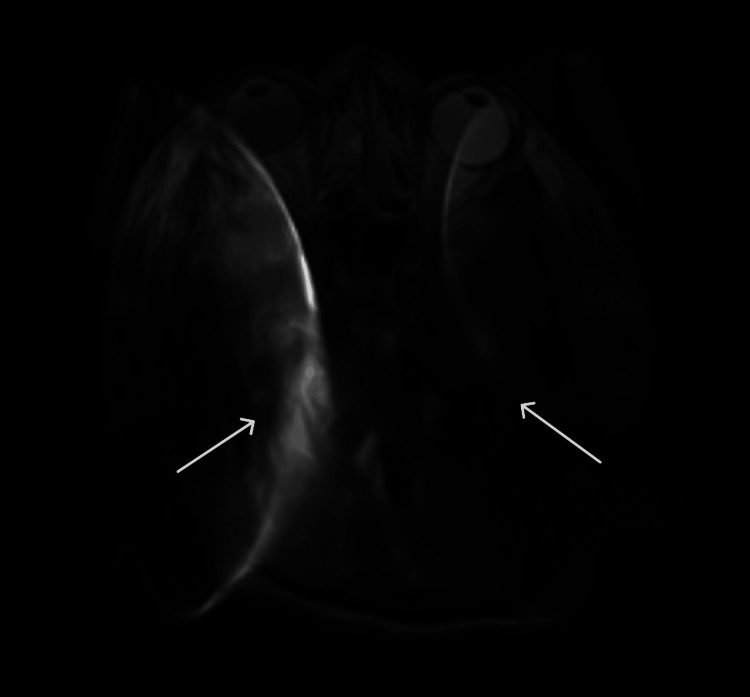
Axial T2-FLAIR MRI of the brain without contrast demonstrating signal artifact from bilateral cochlear implants. Arrows point to significant artifact resulting from the magnetic interaction of the bilateral cochlear implants. T2-FLAIR: T2-weighted fluid-attenuated inversion recovery; MRI: magnetic resonance imaging
